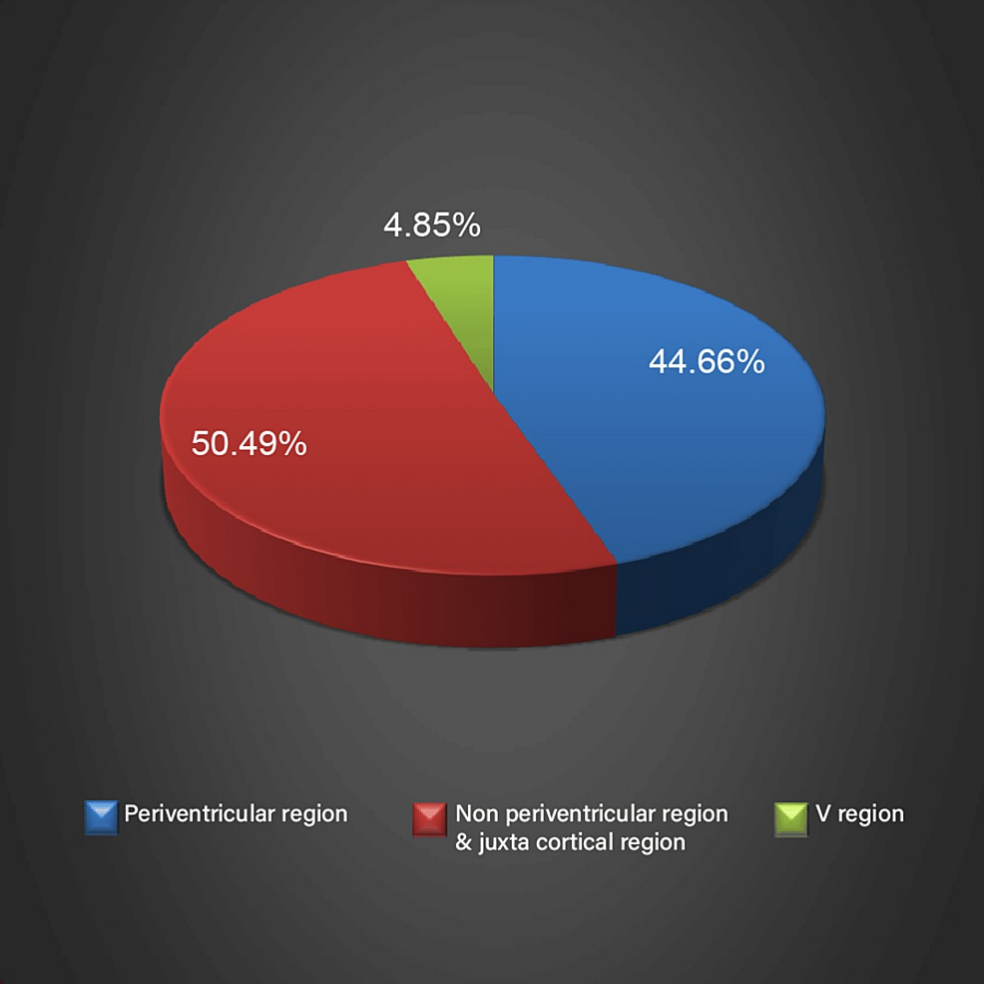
Distribution of lesions on 2D-FLAIR
